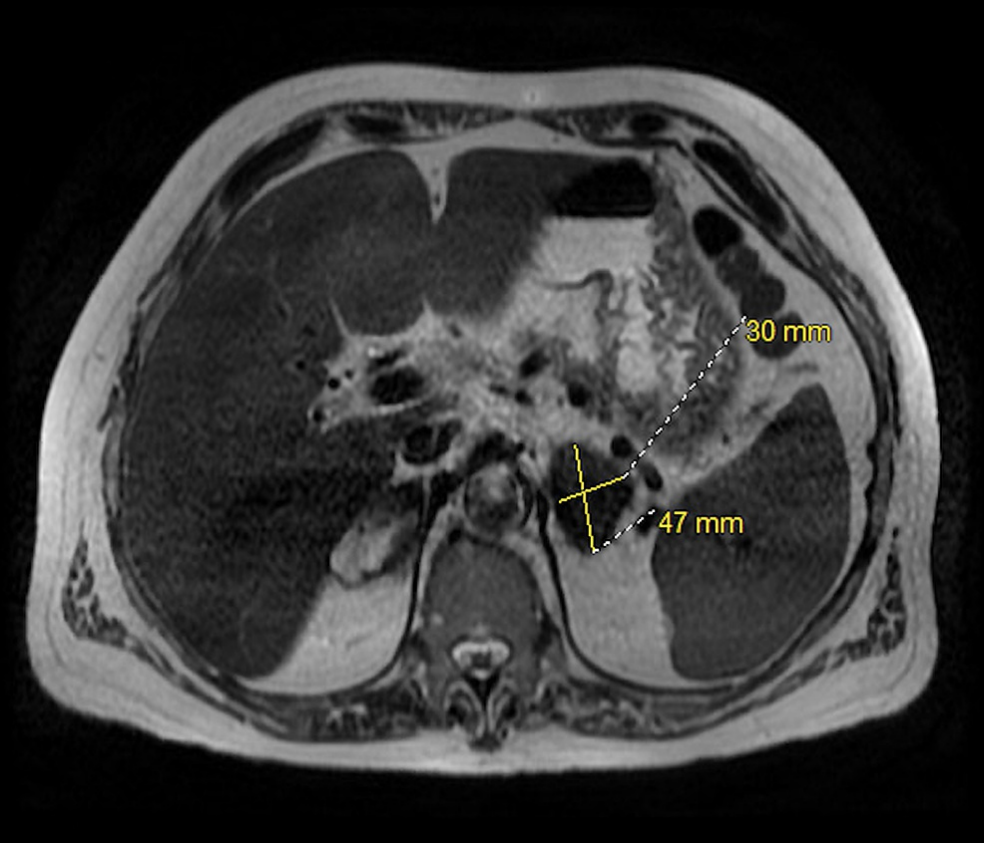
Transversal T1-weighted image of the abdomen without contrast Transversal T1-weighted image of the abdomen without contrast showing left adrenal mass measuring 4.7 cm x 3.0 cm
